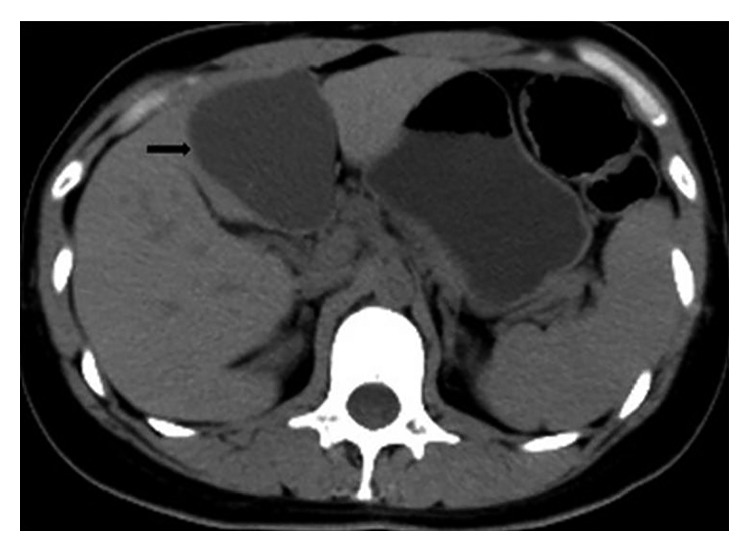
Computed tomography demonstrating a 6.5 × 6 cm endometriotic lesion in the left hepatic lobe.
